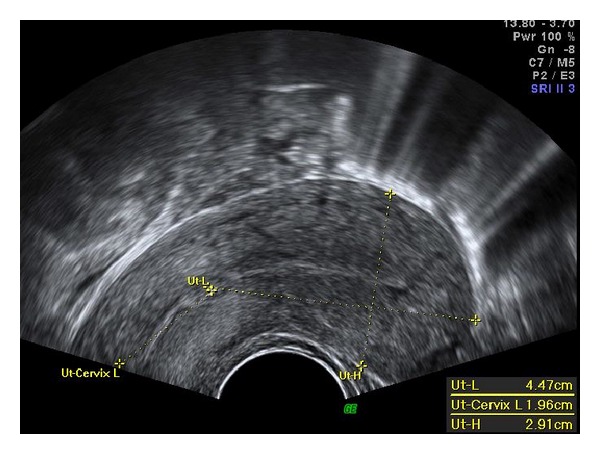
Follow-up endovaginal ultrasound, 3 months later: linear endocervical epithelium.
